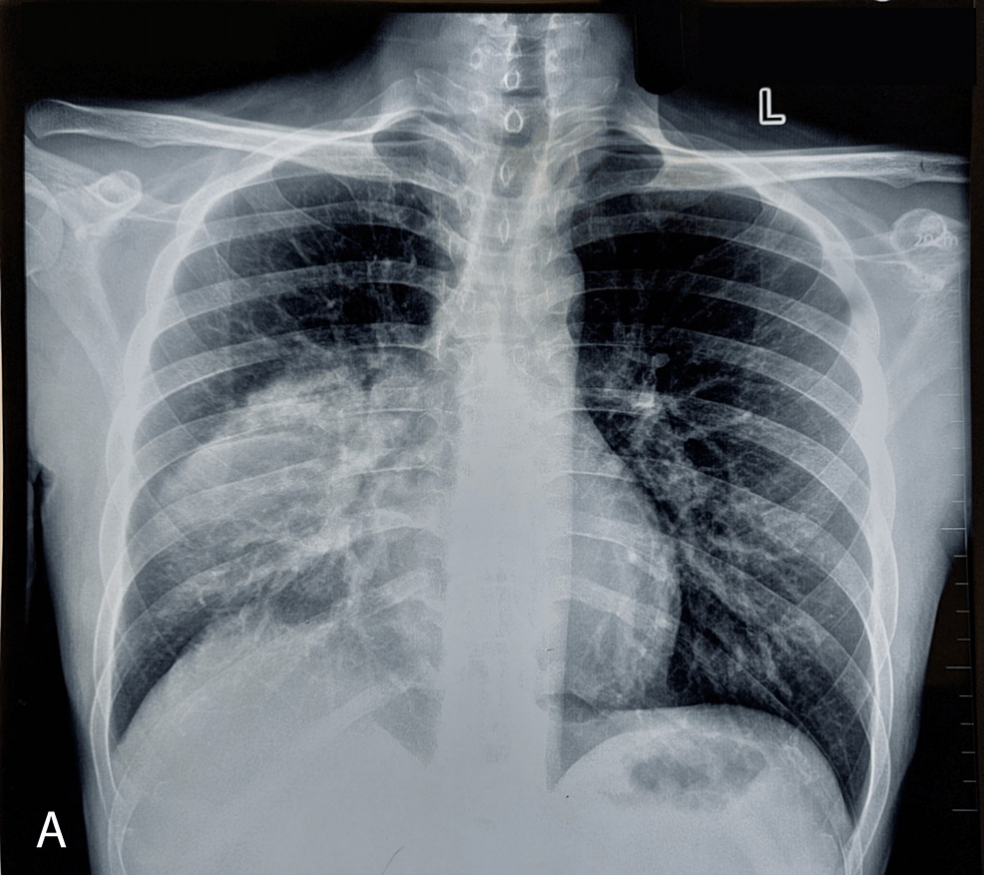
Initial chest X-ray (day 1).
